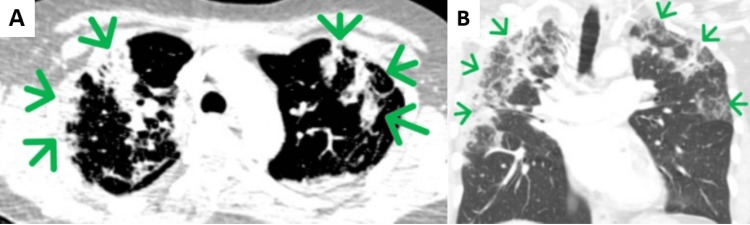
High-resolution CT scan of the chest. Arrows show areas of peripheral consolidation in both axial (A) and coronal (B) views.
